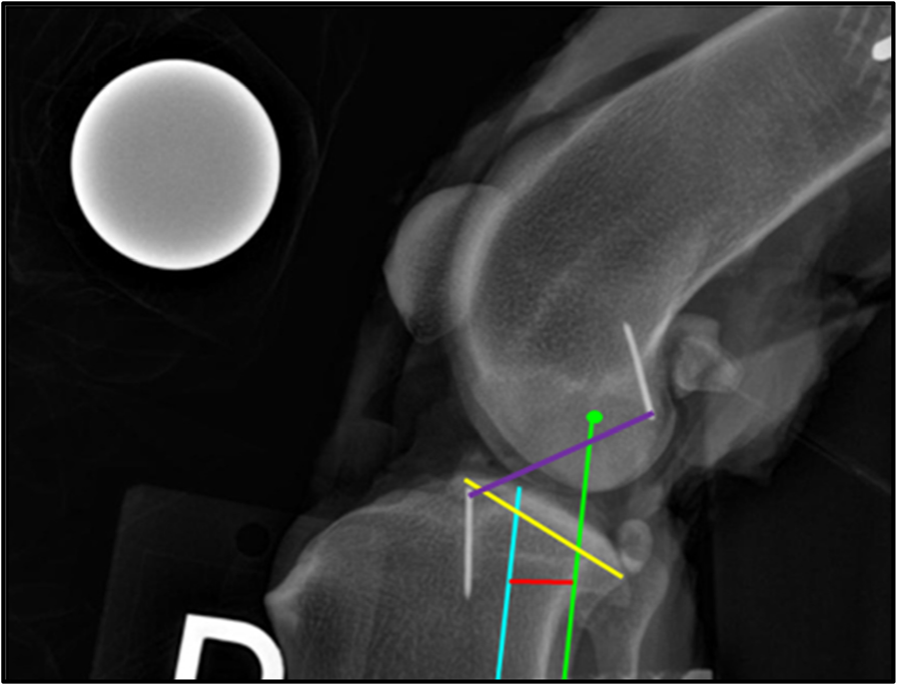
Mediolateral projection radiograph of stifle at 110° of flexion with ICD measurement identified. Purple line: CrCL length (mm), Green dot: center of a best fit circle around the femoral condyles, Blue line: tibial mechanical axis, Green line: femoral condylar axis, Red line: ICD length (mm), Yellow line: tibial medial condyle length (mm)
